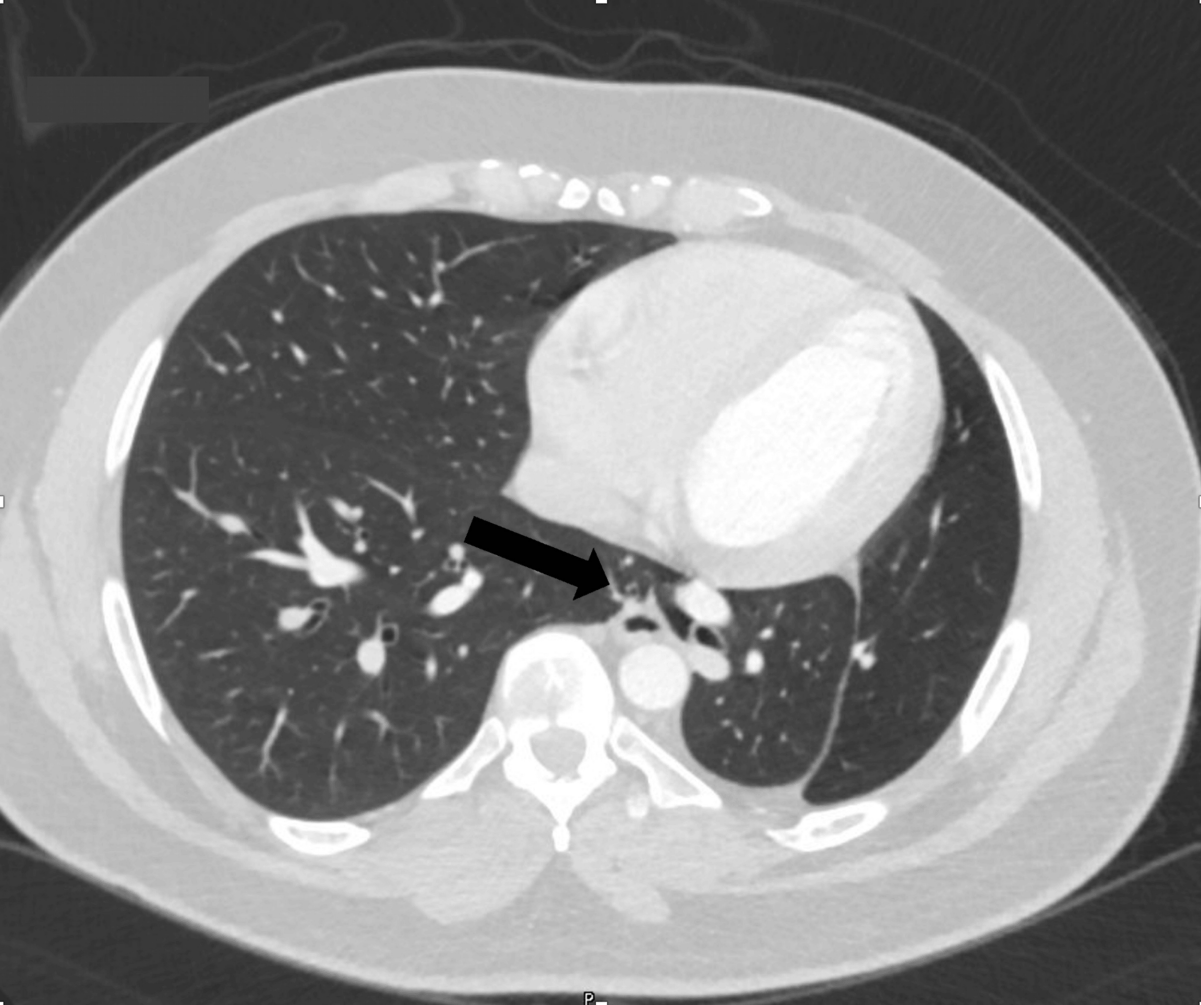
CT chest demonstrates reduced left lung volume with herniation of the right lung into the left hemithorax, positioned behind the heart and anterior to the descending aorta (arrow).
